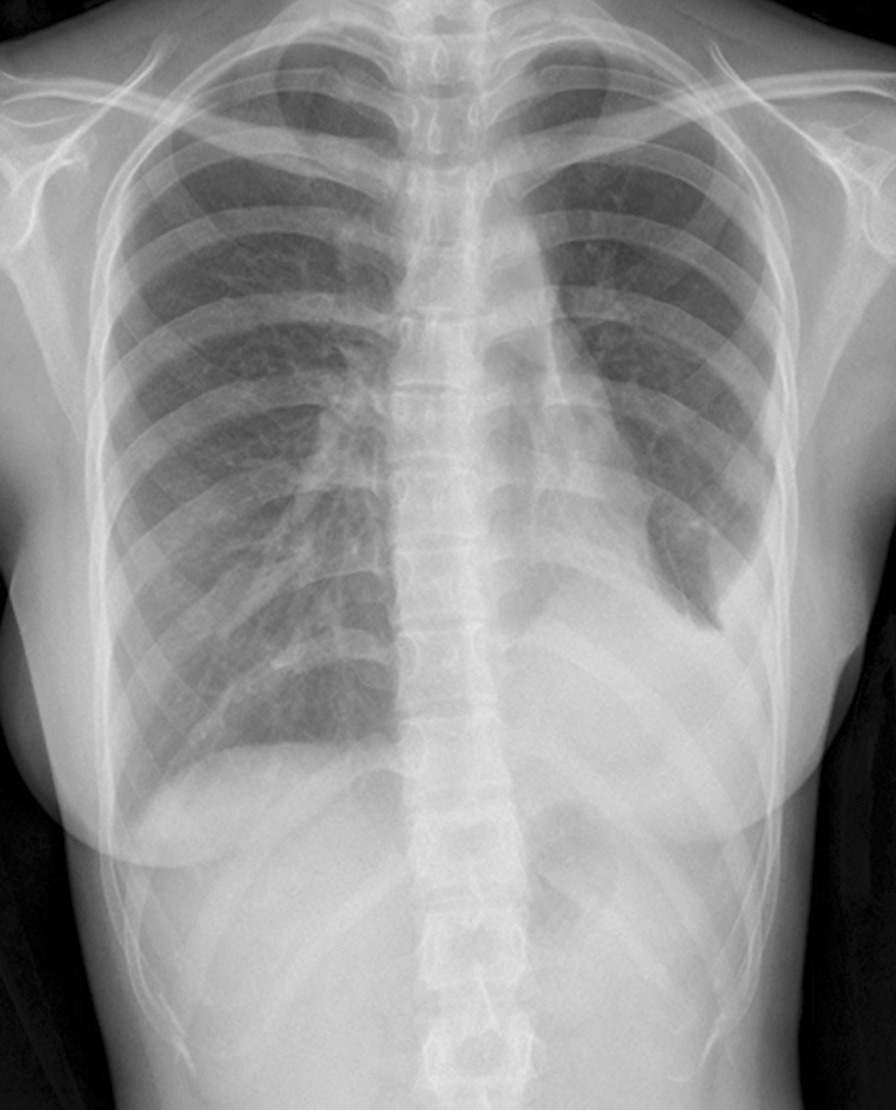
Chest X-ray showing that the left upper lung lobe was completely expanded
